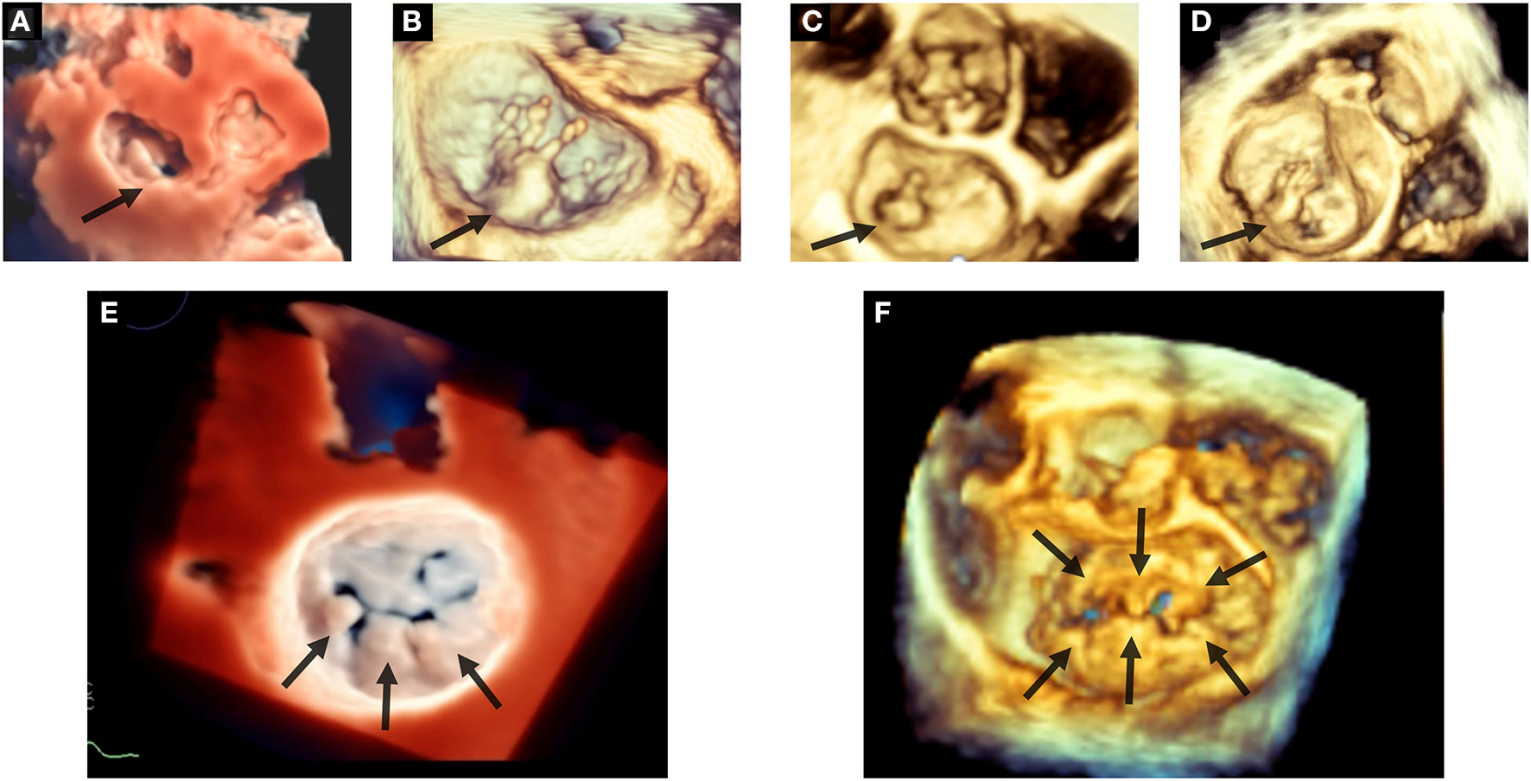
Etiologic phenotypes of mitral valve prolapse. Top panels show examples of fibroelastic deficiency: in (A) P2 prolapse by 3D TTE applying the TI effect; in (B) P2 flail with multiple ruptured chordae by 3D TEE; in (C) P2 flail with single chordal rupture by 3D TEE; in (D) P2 flail with multiple chordal ruptures by 3D TEE. Bottom panels show examples of Barlow's disease: in (E) prolapse of P1–P2–P3 (arrows) by 3D TEE applying the TI effect; in (F) bileaflet prolapse (arrows) by 3D TEE. 3D, three-dimensional; TEE, transesophageal echocardiography; TI, transillumination. TTE, transthoracic echocardiography.
